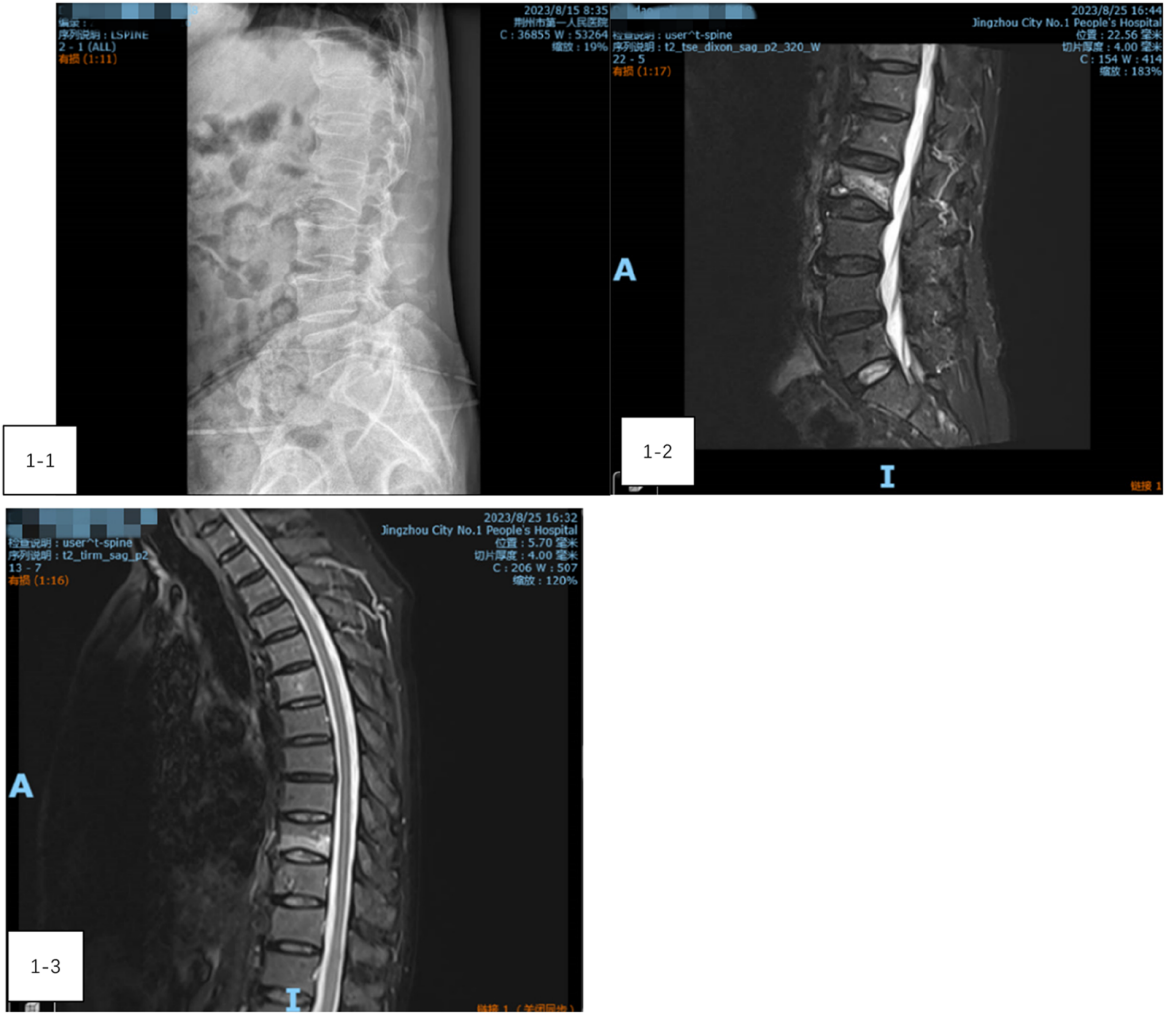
(1-1) A lumbar x-ray confirmed a compression fracture of the L2 vertebra. (1-2) A lumbar MRI further revealed a compression fracture at L2. (1-3) Thoracic vertebra MRI showing bone edema at the T9 vertebra.
